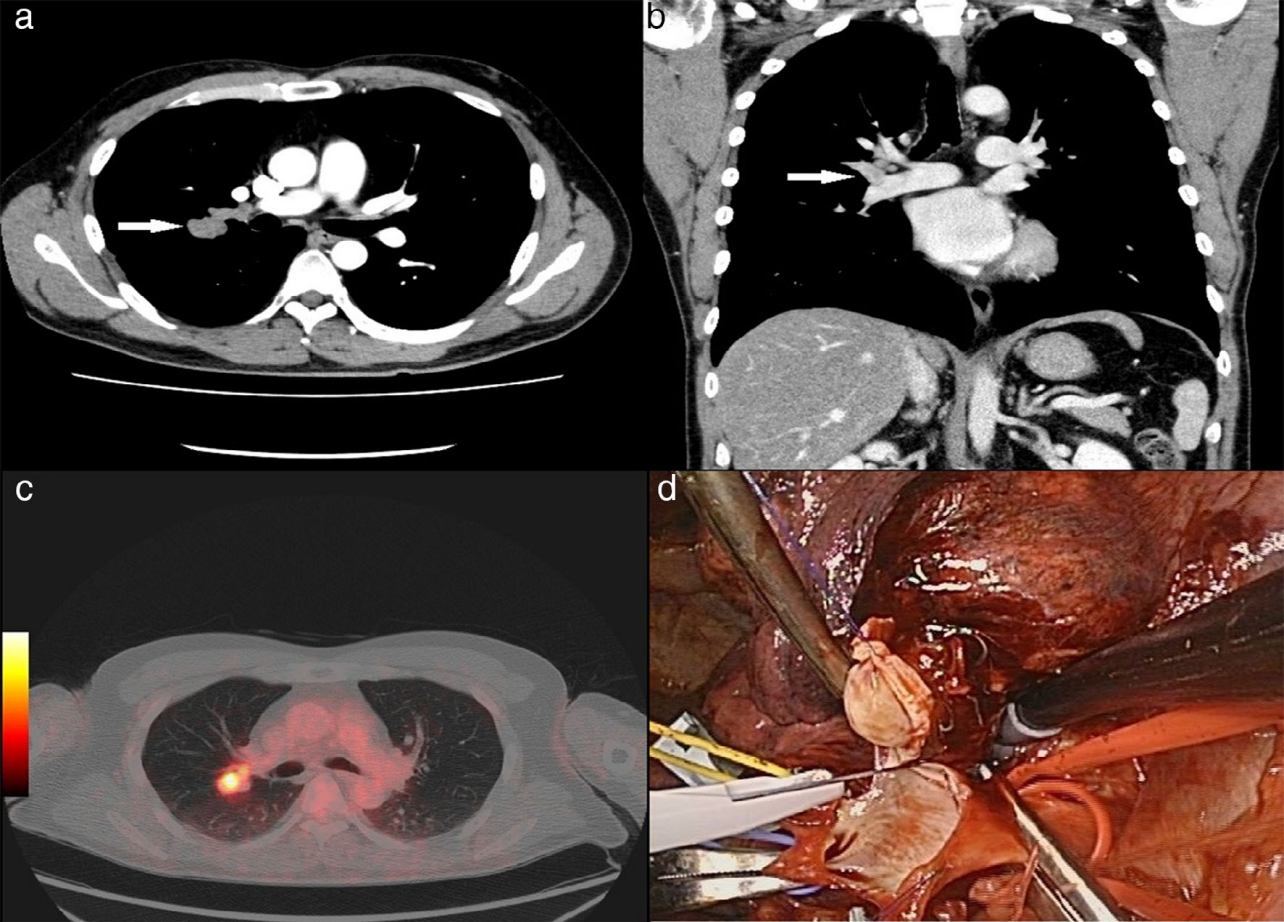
(a), (b) Enhanced chest CT showed an intraluminal mass in the brunch of right pulmonary artery (arrowhead). (c) PET/CT scan revealed significant ^18^FDG uptake, the FEVmax was 7.8. (d) Right upper lobectomy with angioplasty was undertaken
